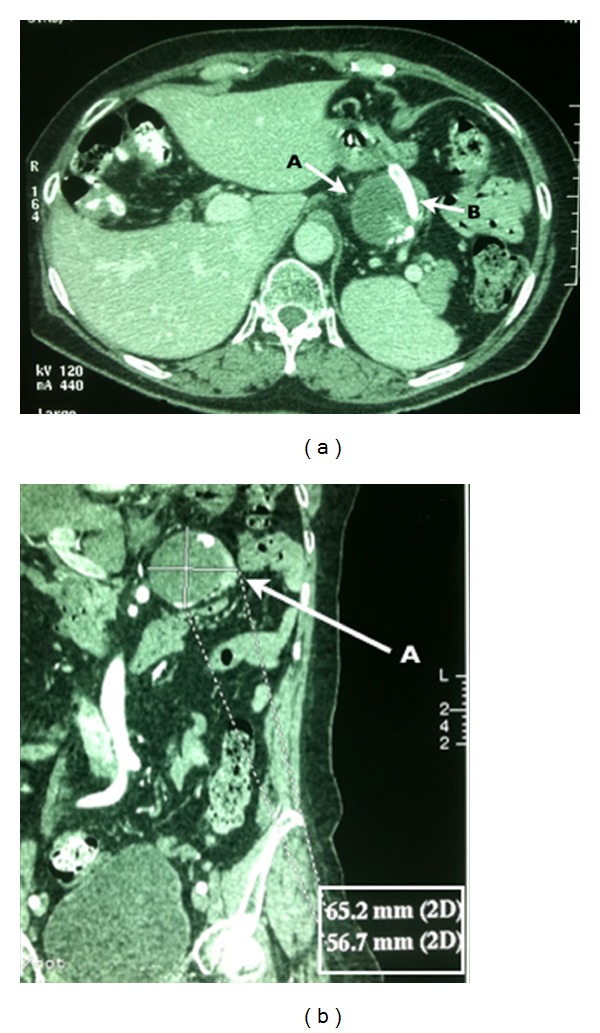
Computed tomography of the abdomen with intravenous contrast (postoperative control). (a) Axial section: A: thrombosed aneurysm sac, B: flow of contrast through the implanted stent. (b) Coronal section: A: thrombosed aneurysm sac, highlighted at the bottom of the figure, the measures of the aneurysm.
